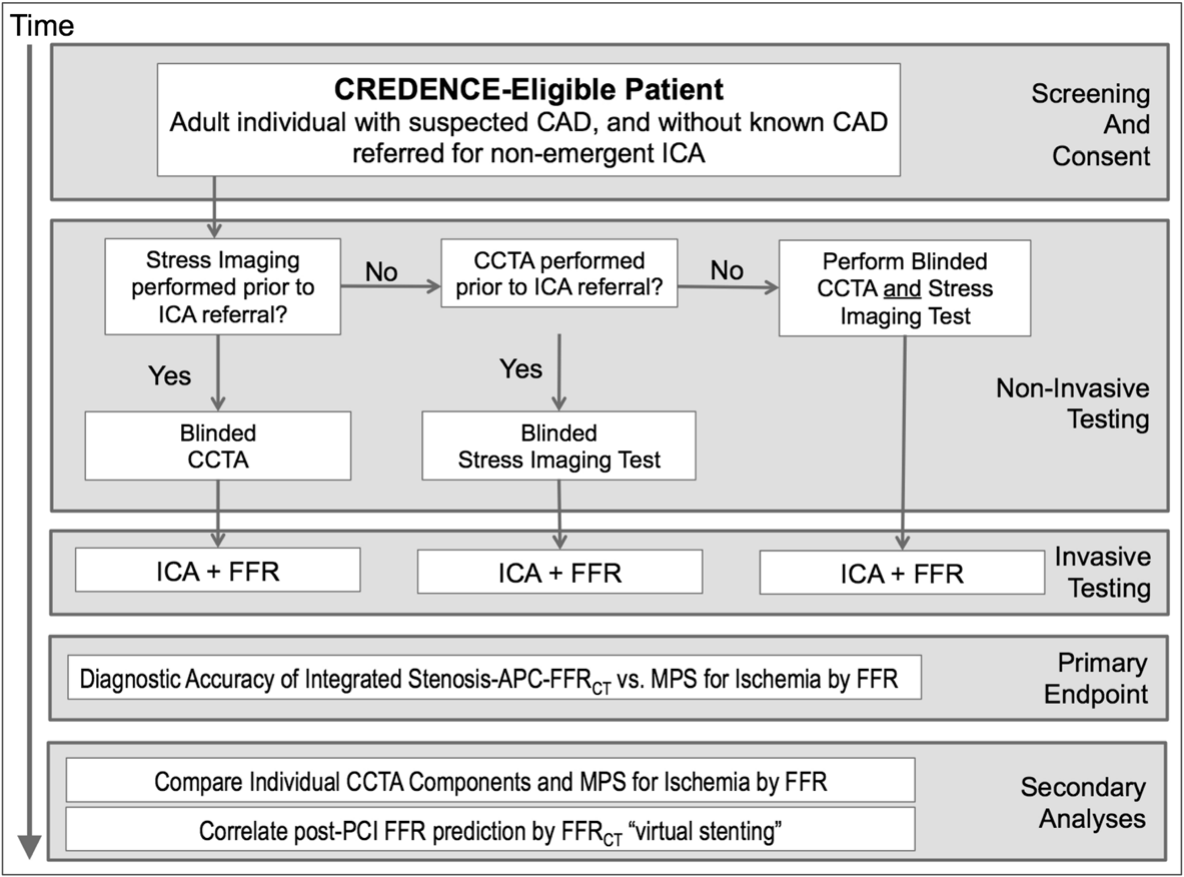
CREDENCE study design. CAD, coronary artery disease; ICA, invasive coronary angiography; CCTA, coronary computed tomography angiography; MPS, myocardial perfusion scintigraphy; FFR, fractional flow reserve; FFR_CT_, fractional flow reserve derived from coronary computed tomography angiography; APCs, atherosclerotic plaque characteristics; PCI, percutaneous coronary intervention
